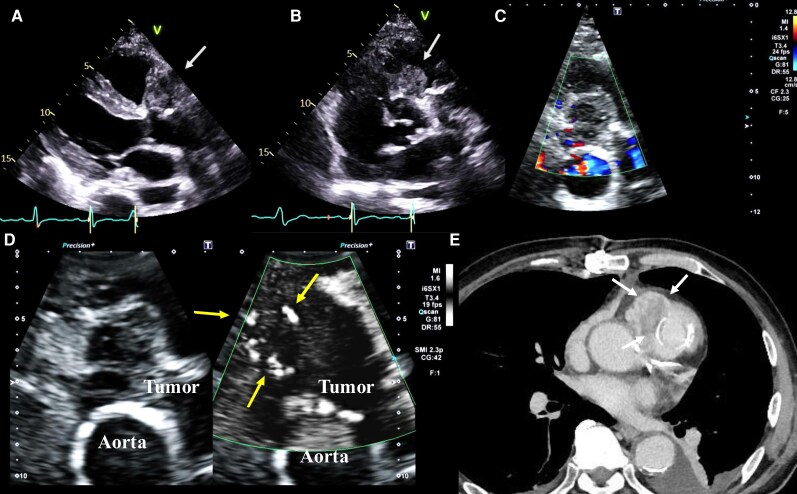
A case of pulmonary artery intimal sarcoma. Transthoracic echocardiography scans in a parasternal view (*A*) and a short-axis view (*B*) show a residual tumour measuring 31 × 32 mm in the right ventricular outflow tract (white arrows). (*C*) The intratumoural blood flow signal could not be clearly detected by conventional colour Doppler, even with a reduced velocity scale. (*D*) Superb microvascular imaging detected abundant intratumoural blood flow signals (yellow arrows). (*E*) Cardiac contrast-enhanced computed tomography scan showing a residual tumour with an irregular margin and a surrounding contrast effect in the right ventricular outflow tract (white arrows).
